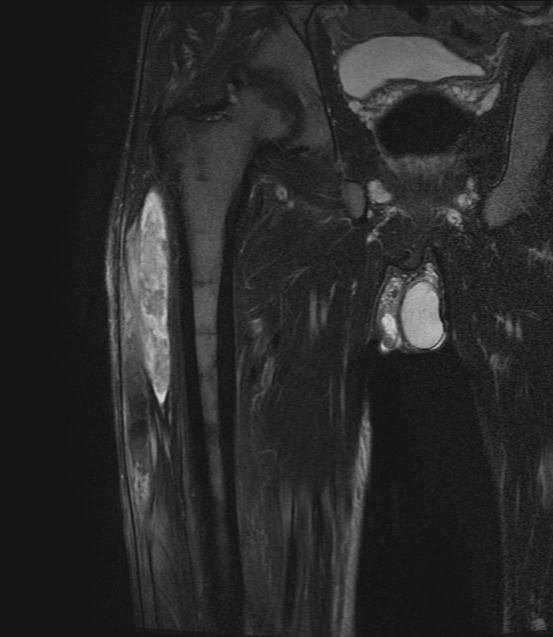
Coronal T2-weighted fat-saturated sequence shows the cranio-caudal extension of the hematoma in the thigh.
